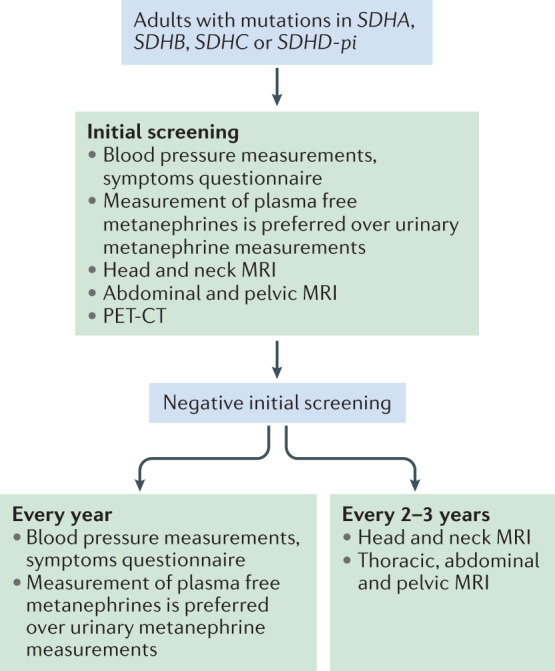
Screening and follow-up proposed during adulthood. An initial tumour screening should be performed after the discovery of an *SDHA*, *SDHB*, *SDHC* and *SDHD-pi* mutation relying on blood pressure measurements, a symptoms or signs questionnaire, assessment of metanephrines in plasma or urine, and imaging work-up by MRI of head and neck, abdomen, pelvis, and a whole-body PET–CT. Even after an initial negative work-up, all asymptomatic mutation carriers should be followed clinically and by biochemistry assessments every year and by MRI every 2–3 years. Thoracic MRI is not mandatory at the first initial work-up if PET–CT does not show any abnormality but is recommended for subsequent follow-up.
